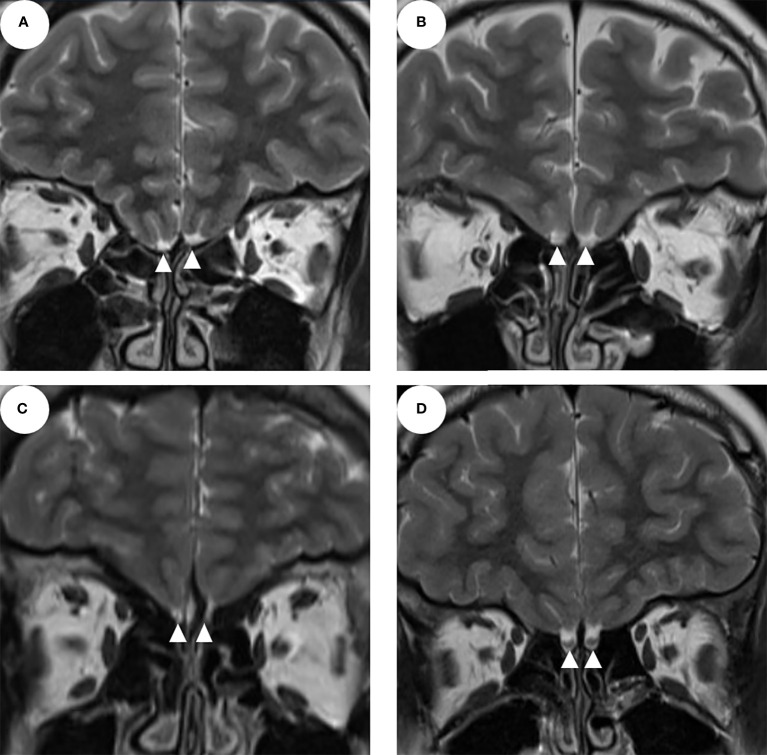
The MRI of the olfactory apparatus (A–C show the bilateral olfactory bulb and/or sulcus aplasia; D shows normal olfactory bulbs and sulcus; the white triangles indicate the olfactory bulbs).
